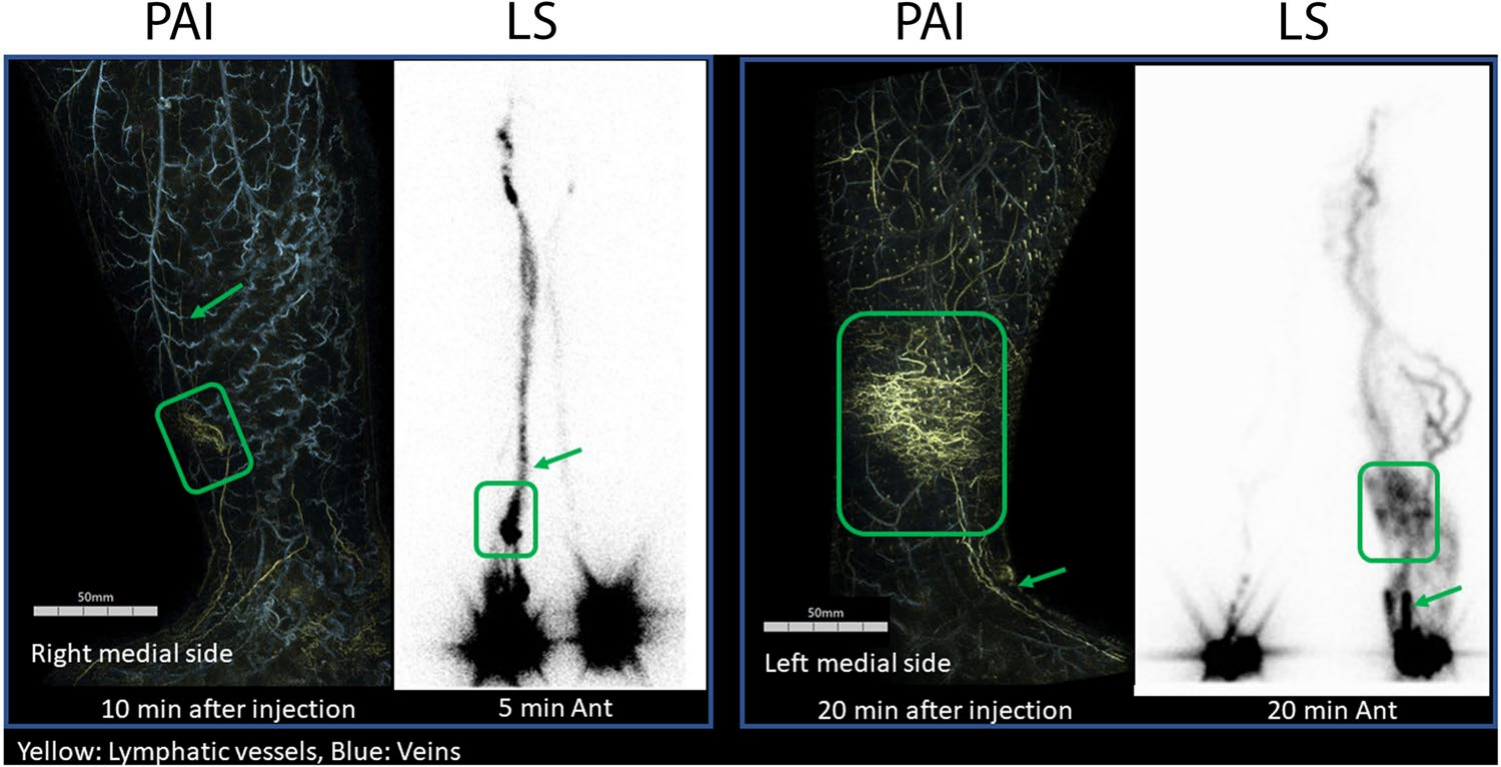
Comparative images between traditional lymphoscintigraphy (LS) and photoacoustic imaging (PAI). Dermal backflow (DBF) was visualized similarly using both techniques. Both show representative cases of type 3 lymphedema with dermal back flow in the early phase of lymphoscintigraphy. Each DBF is surrounded by a *square*, and the linear lymphatic vessels proximal and distal to it (*arrows*) can be seen in both examinations. (Adapted with permission from Watanabe et al., 2022, ©2022, Journal of Vascular Surgery: Venous Lymphatic Disorders, published by Elsevier)
